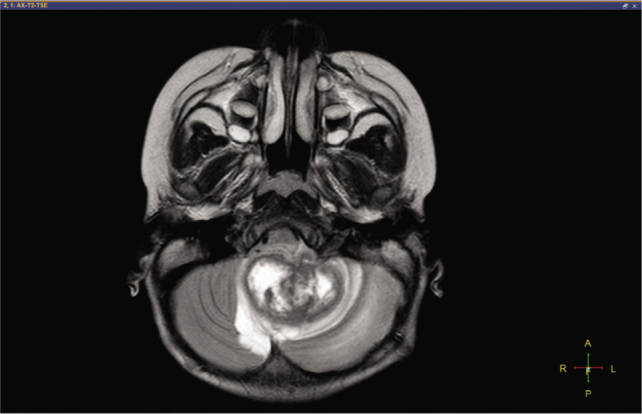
Axial T2-weighted image shows a predominantly cystic mass located in the fourth ventricle.
